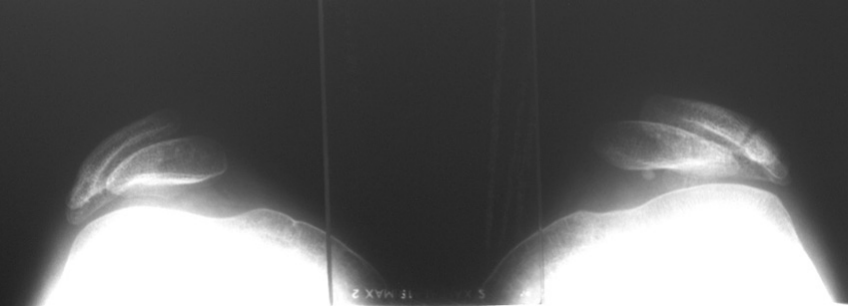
Preoperative x-ray (skyline view (60° flexion)) of both knees demonstrating subluxation of both patellae.
